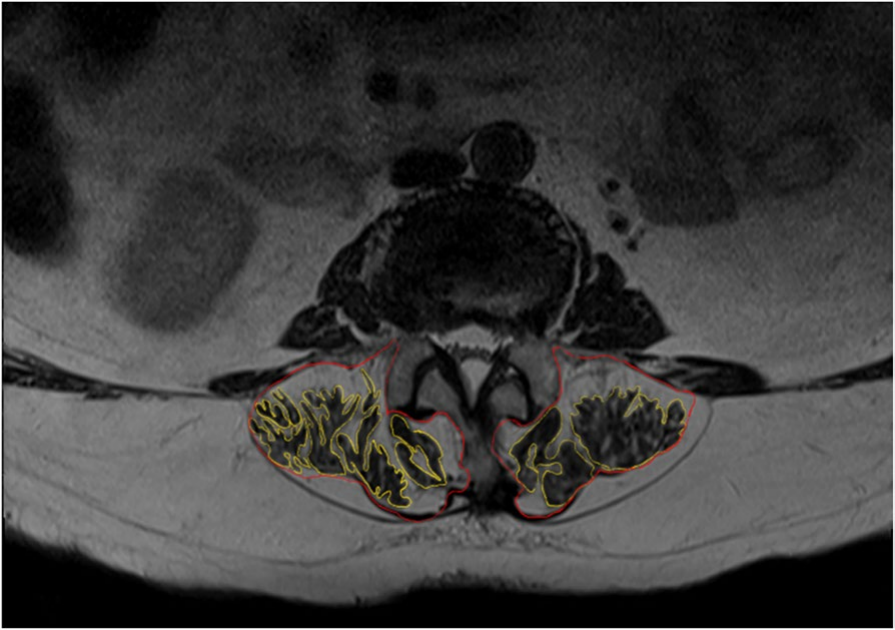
MRI performed to evaluate the physiological cross-sectional area of the PVM
